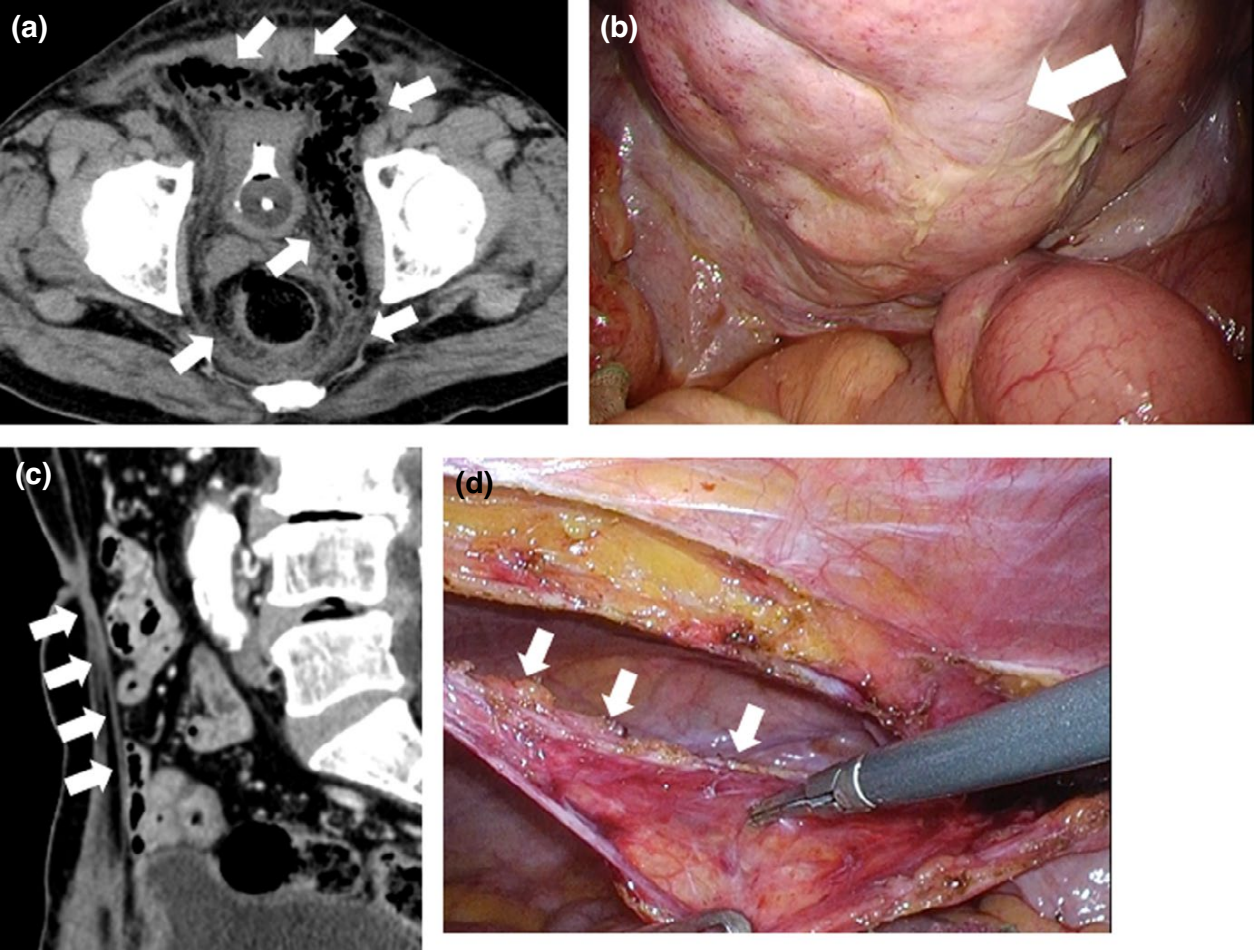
(A) CT. Gas and extensive abscess formation in the preperitoneal space from the lower abdomen to the pelvis. (B) Laparoscopic image. There was marked swelling of the abdominal wall centered on the midline umbilical cord, suggesting abscess formation in the same area. (C) CT. A slightly hyperdense cord‐like structure extending from the umbilical region to the bladder, which was assumed to be a urachal remnant. (D) Laparoscopic image. The median cord, i.e., the presumed urachal remnant, was detached from the abdominal wall
